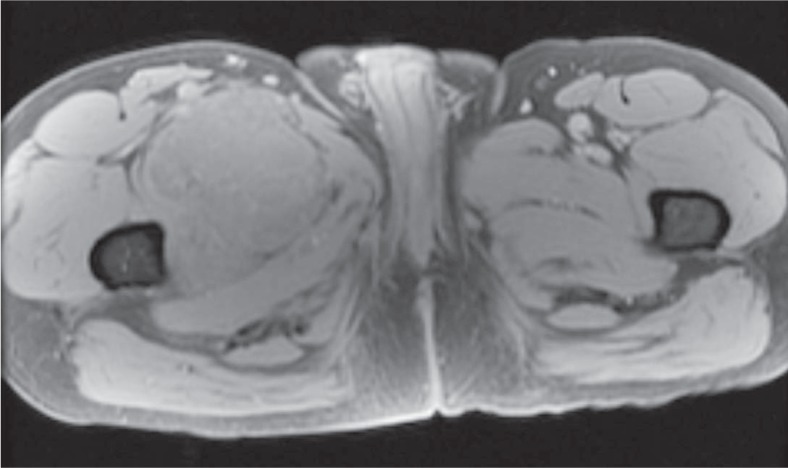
Axial T1 fast spoiled gradient-echo (FSPGR) FS pre-contrast MRI shows that this tumor is isointenese to muscle on T1 weighted images.
